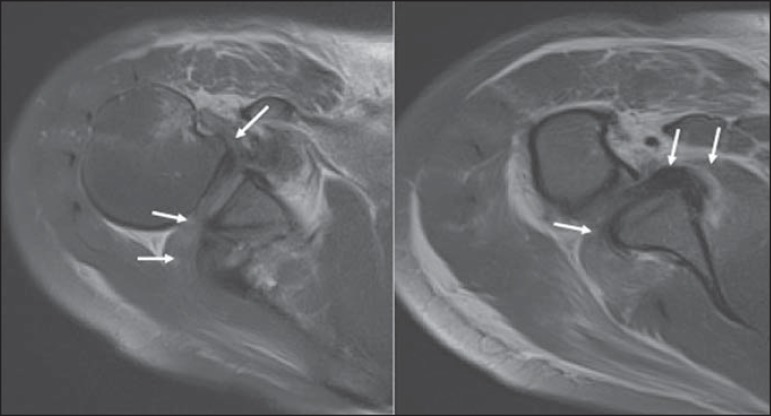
MRI. Axial sections images acquired with intermediate weighting
